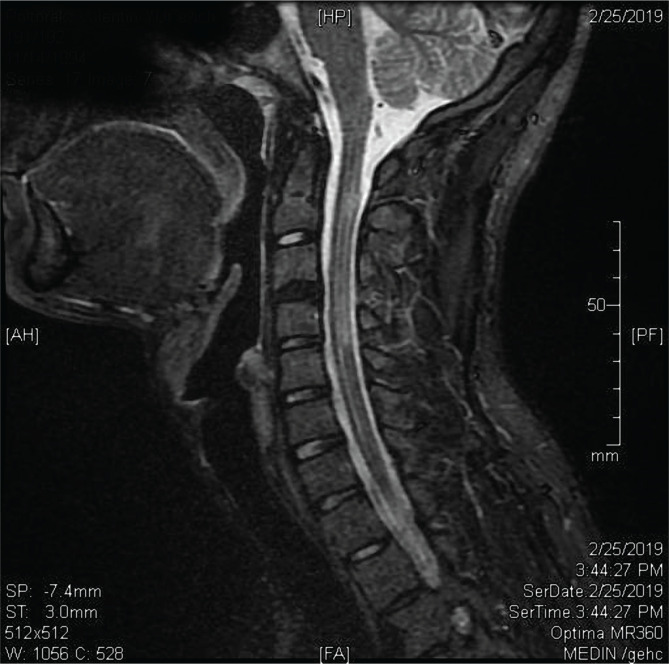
Sagittal T2-weighted MRI sequence of the cervical spinal cord revealing nonhomogeneous cervical spinal cord with a focal area of centro-posterior myelopathy (C1-C2: 3×4×15 mm), non-enhancing (white arrows).
